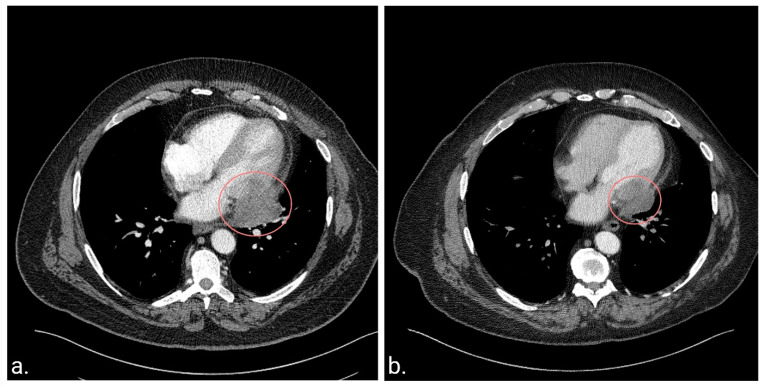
Chest computerized tomography (CT) scans at diagnosis of the cardiac metastasis and 10 years post-treatment. (a). CT scan before the initiation of chemotherapy and radiotherapy demonstrating a heterogeneous mass located in the pericardium and extending into the left ventricle. (b). The latest CT scan 10.5 years after diagnosis demonstrating a residual soft tissue mass centered in the left posterolateral pericardium, which has remained stable since the completion of therapy.
